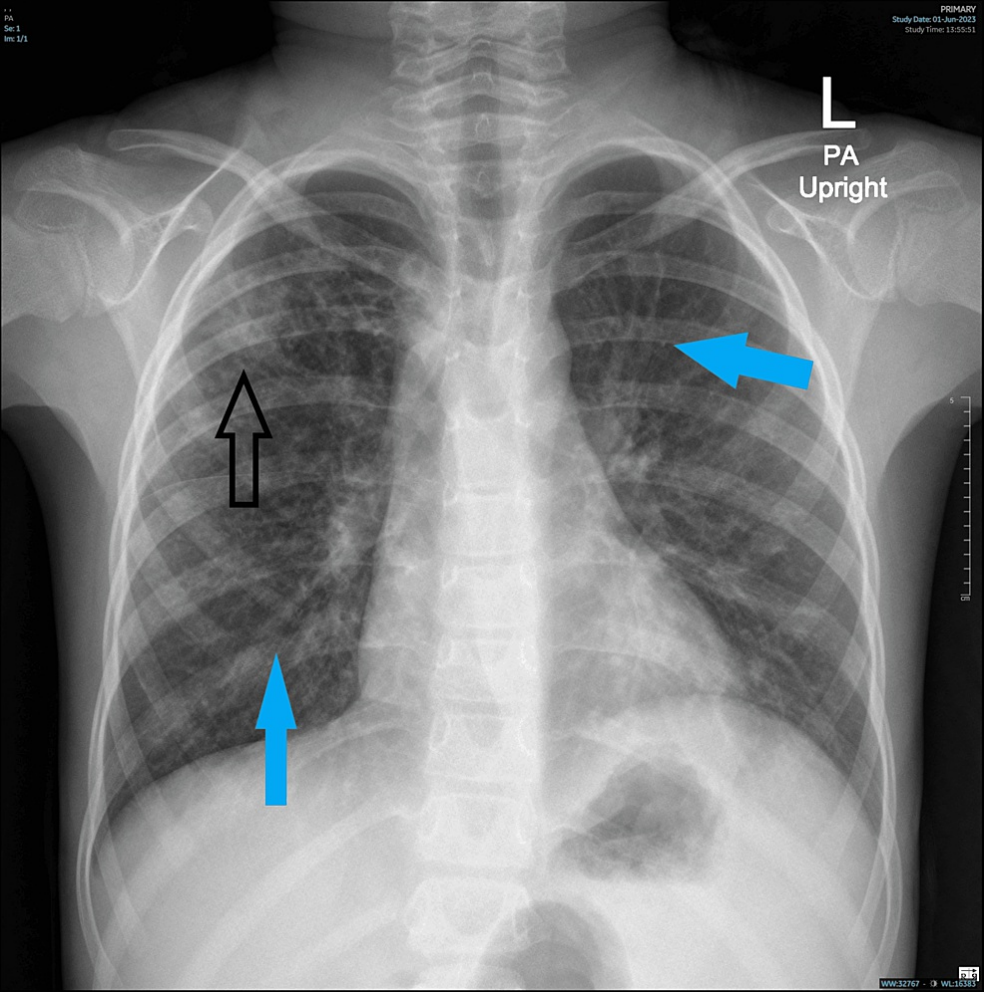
Chest X-ray image The chest X-ray showed patchy air space opacity in the right upper lung zone, likely infective in nature (black arrow). Bilateral accentuated broncho-vascular marking with minimal peri-bronchial thickening (blue arrow).
